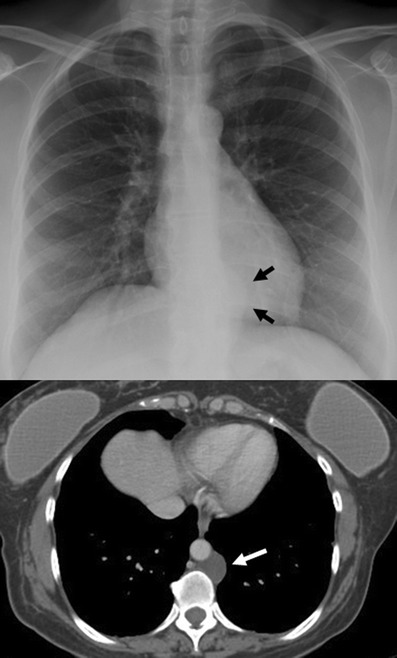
A cystic lymphangioma (also referred to as hygroma) in a 47-year-old woman with retroperitoneal disease. A posterior mediastinal mass with a homogeneous fluid-attenuation is identified on CT (*white arrow*) and on posteroanterior chest radiograph as a mass disrupting left paraspinal line inferiorly (*black arrows*)
